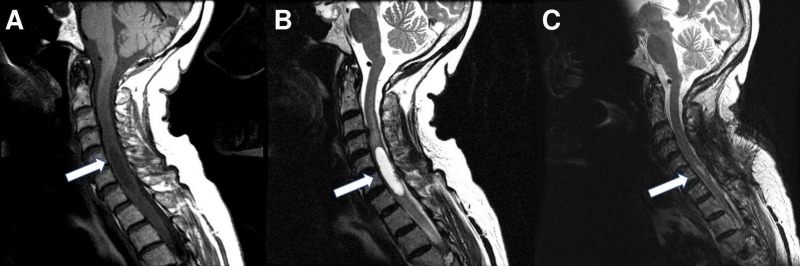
Preoperative and postoperative imaging of cervical intramedullary hemangioblastoma with associated cyst Magnetic resonance (MR) images showing a cervical syrinx and nodule on (A) preoperative sagittal T1-spin echo (SE) and (B) T2-fast recovery fast spin echo (FRFSE). (C) Postoperative sagittal T2 MR image after removal of the hemangioblastoma with decompression of the syrinx.
